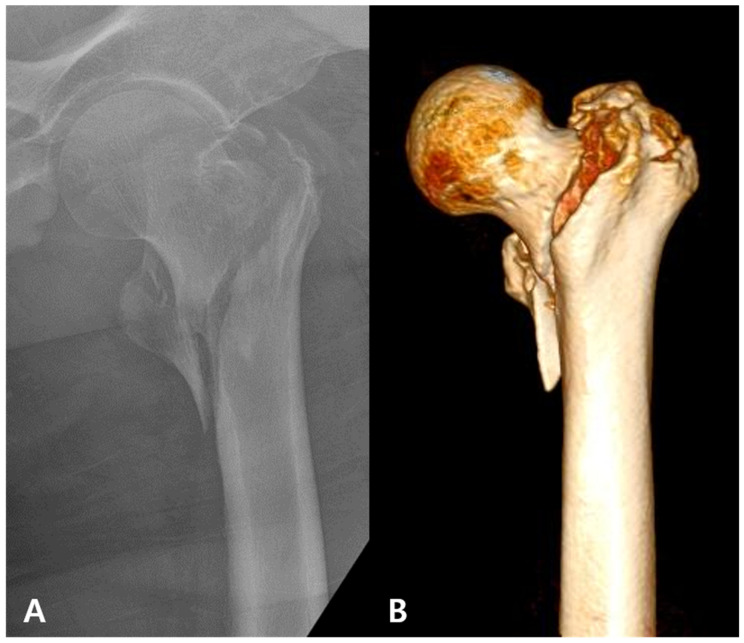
Anteroposterior radiograph (A) and 3-D CT image (B) showing unstable basicervical IT fracture of the left proximal femur.
